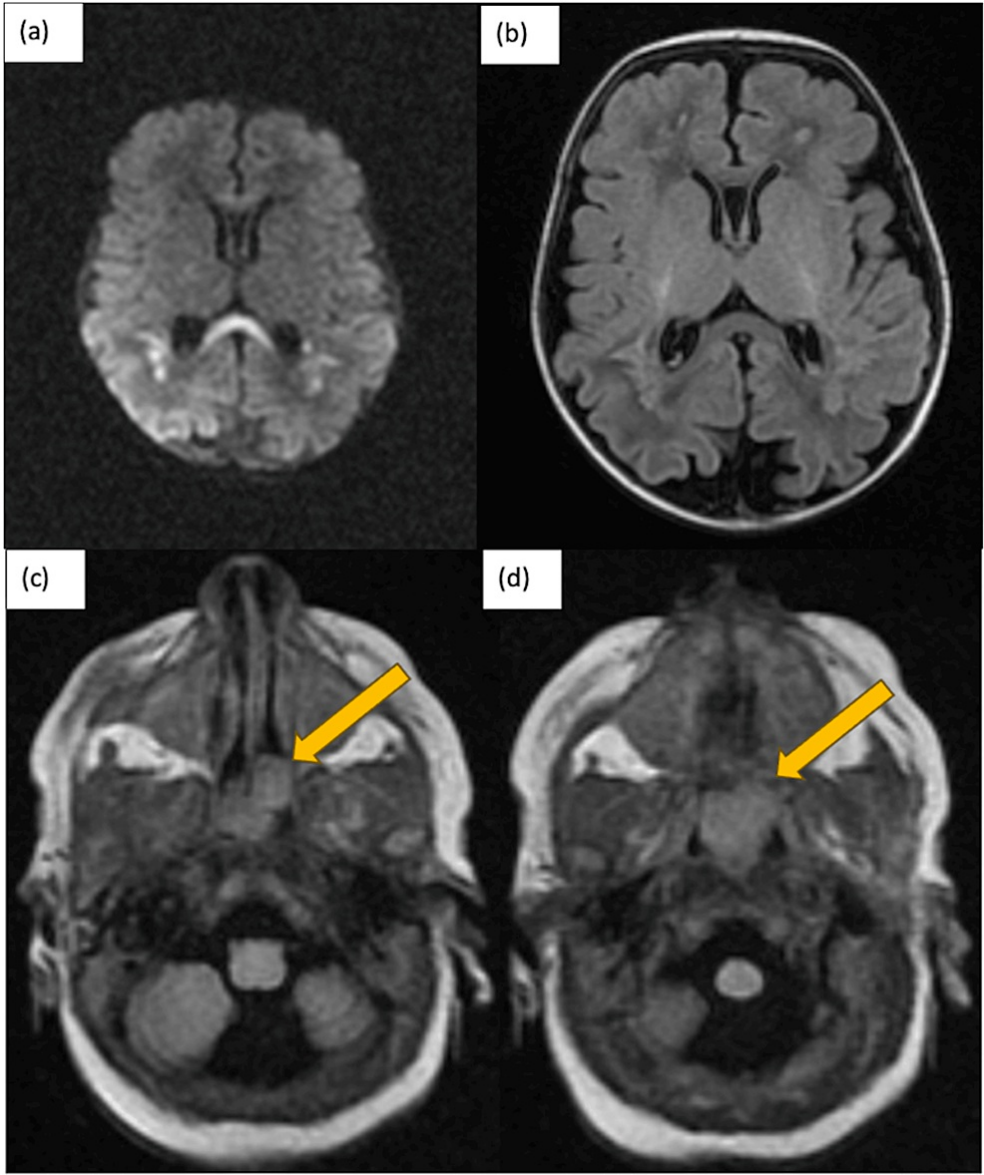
Initial brain MRI done on day of life 10. (a, b) Neurologic radiographic findings of the diffusion abnormality of the corpus callosum and posterior periventricular white matter compatible with HIE. Additional patchy T1/T2 signal abnormality of the bilateral deep white matter without corresponding diffusion abnormality likely periventricular leukomalacia (PVL)/hypoxic ischemic encephalopathy (HIE). (c, d) Findings of the nonspecific polypoid soft tissue partially filling the nasopharynx with no definite evidence of osseous choanal atresia.
